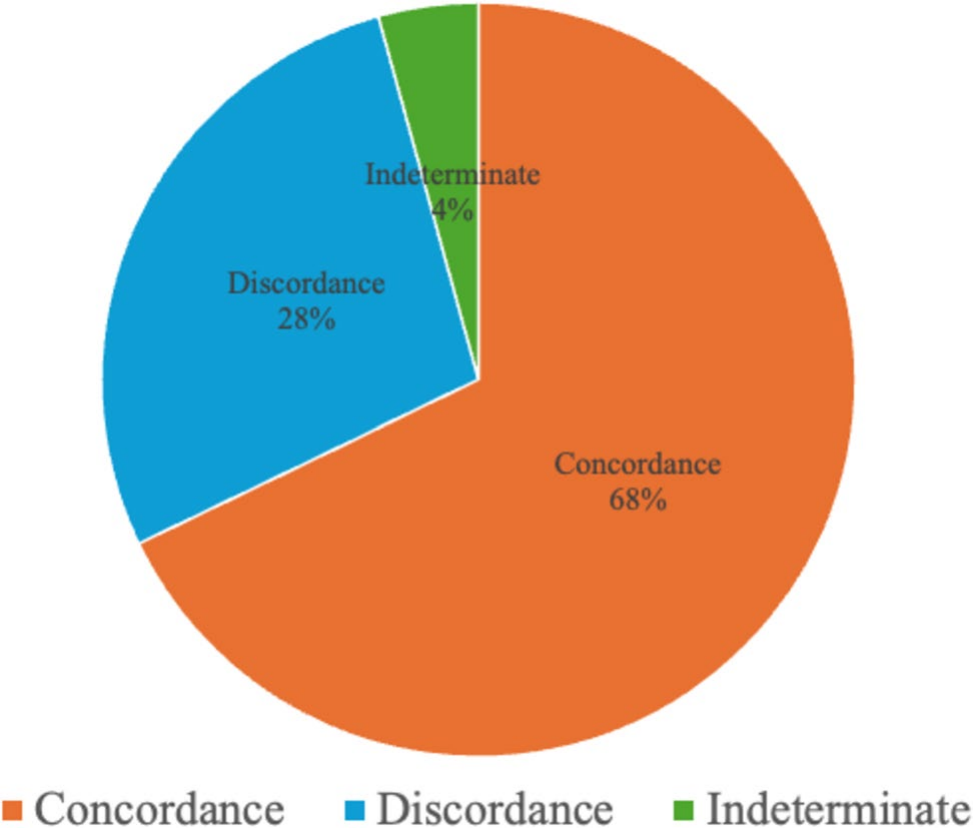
Distribution of concordance classifications between CEM and DCE-MRI on a per-patient basis. Among the evaluated cases, 68% demonstrated complete concordance (orange), 28% showed clinically relevant discordance (light blue), and 4% were deemed non-evaluable or indeterminate (green)
